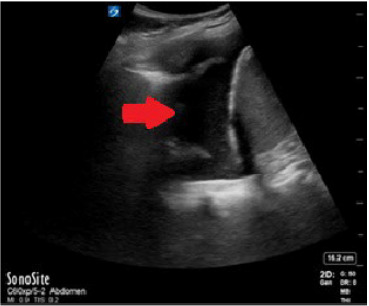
Point-of-care thoracic ultrasound showing a right-sided pleural effusion with the diaphragm in view. Arrow indicates pleural effusion.
